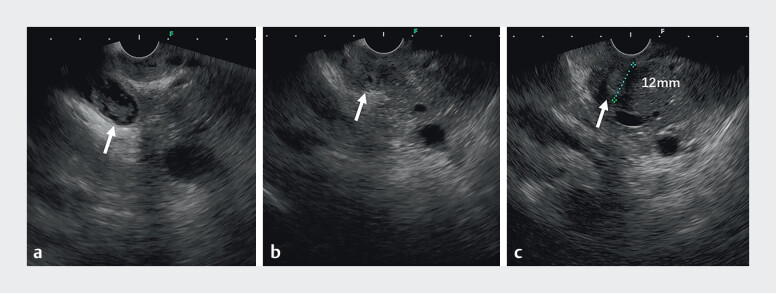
Endoscopic ultrasonography findings indicating an ectopic duodenal papilla.
